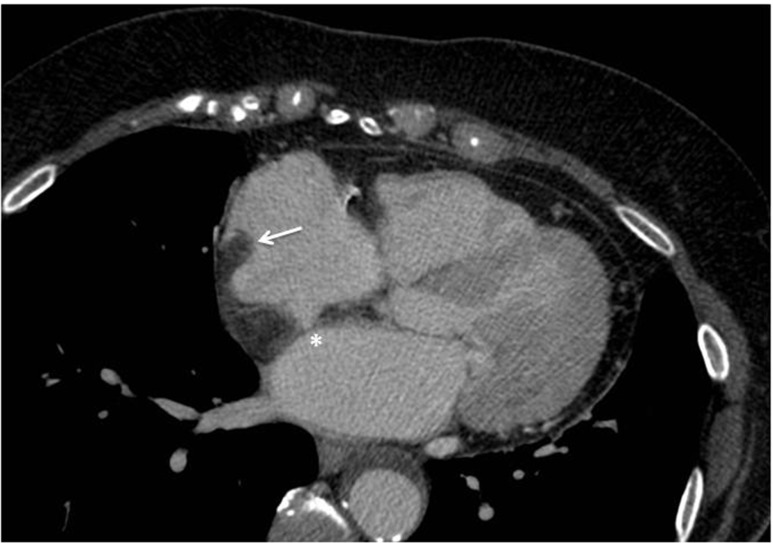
Lipomatous hypertrophy of the interatrial septum. Cardiac CT demonstrating a mass with fat-attenuation which spares the fossa ovalis (*) consistent with lipomatous hypertrophy of the interatrial septum. Note that the mass also extends to involve the posterior wall of the right atrium and crista terminalis (arrow), which may be seen in cases of more extensive lipomatous hypertrophy
